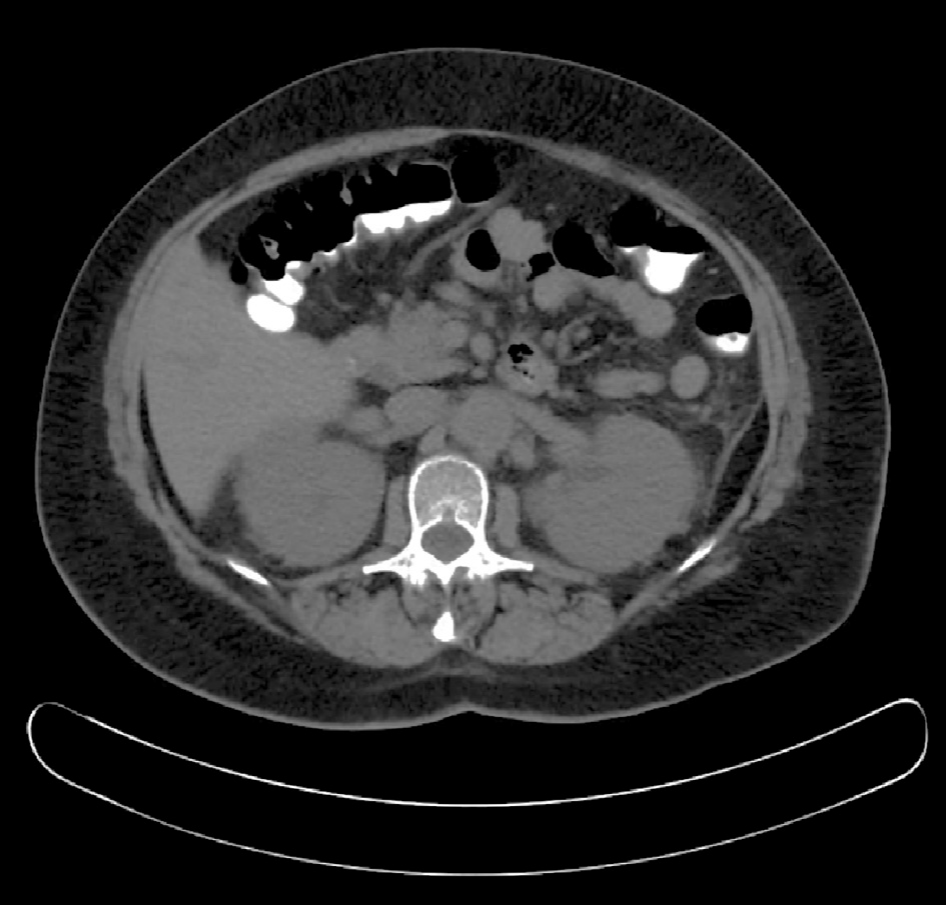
Abdominal CT demonstrating perinephric infiltrate bilaterally, giving a “hairy kidney” appearance.
